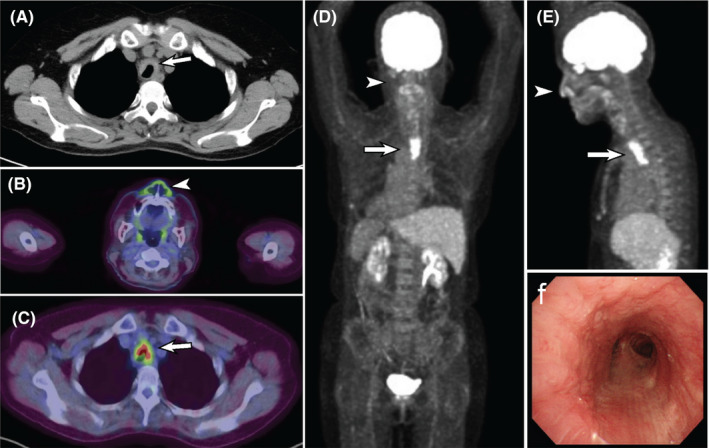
A, Plain CT image of the chest showing tracheal wall thickening, predominantly in the anterior and lateral walls (*arrow*). B‐E, Axial PET‐CT fusion images (B and C) and maximal intensity projection (MIP) images (D and E) showed increased FDG accumulation in the nasal alar cartilages (*arrowheads*) and trachea (*arrows*); the posterior membranous portion of the trachea appears to be uninvolved. F, Fiberoptic bronchoscopic image showing mucosal edema and cartilaginous hypertrophy of the trachea
